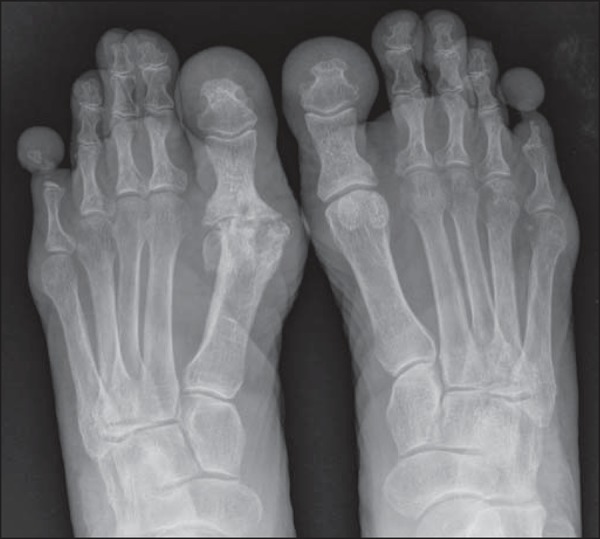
Radiography – anteroposterior view of the feet two years after the symptoms onset. In
